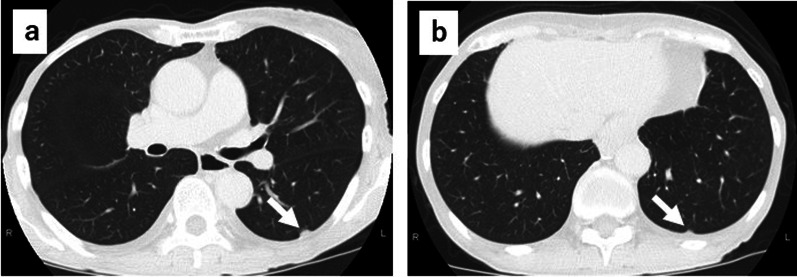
CT images of the chest. a, b CT of the chest, showing nodules in the subpleural area of the lower lobe of the left lung (white arrow)
